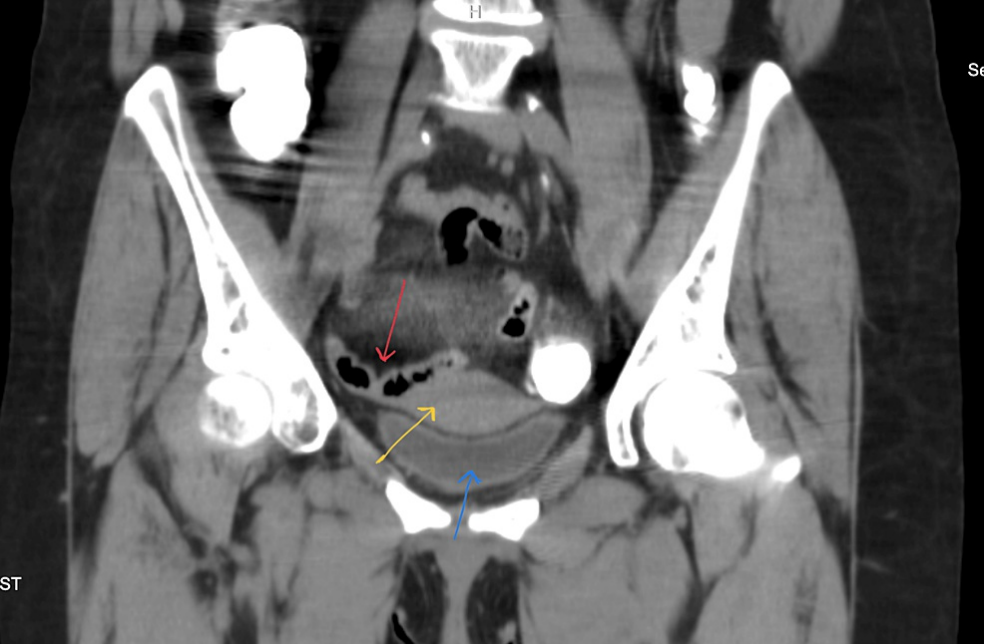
Coronal CT scan with contrast of the pelvis shows the bladder (blue arrow), uterus (yellow arrow), and air in the small bowel loop (red arrow) with inflammatory changes in fat stranding in the middle (haziness). CT: computed tomography
